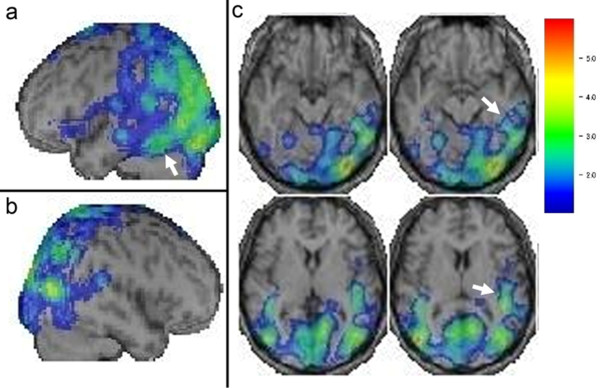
Single-photon emission computed tomography scans evaluated using the easy z-score imaging system (a-c). Hypoperfusion areas including the bilateral parietal and occipital lobes, left temporal lobe, and left posterior inferior temporal lobe (white arrows) are shown. (a) Left lateral view. (b) Right lateral view. (c) Axial views.
